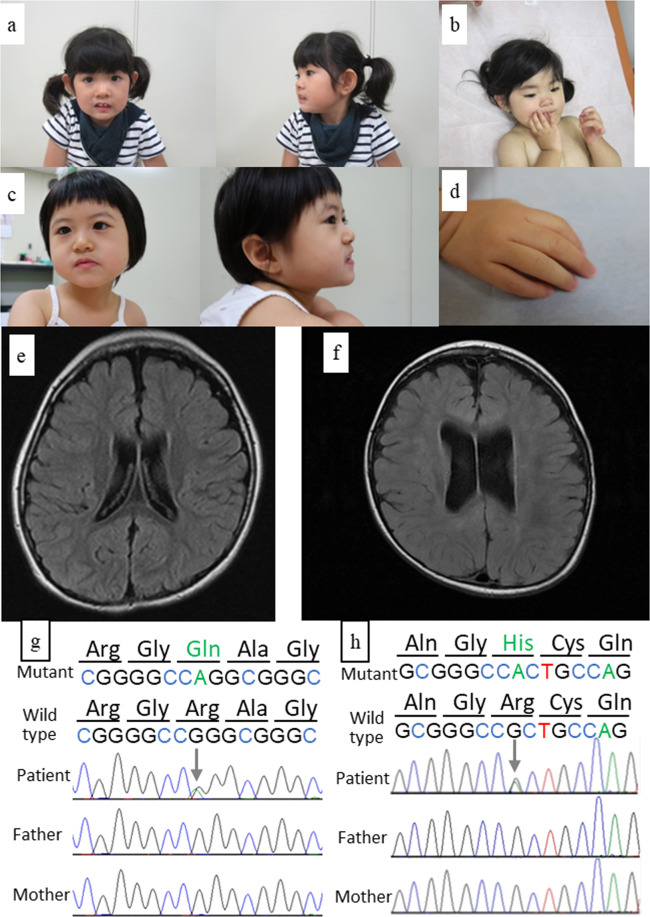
Patients with *DHX30* variants and their MRI findings. a Patient 2 at 3 years of age. The patient exhibited thick low-set ears, long eyelashes, interdental space, high arched palate, micrognathia, b tapered fingers and hand licking. c Patient 3 at 5 years of age. The patient exhibited internal strabismus, epicanthus, thick eye brow, long eyelashes, and d tapered fingers. e Axial T1-weighted brain MRI of Patient 2 demonstrated a decreased white matter volume and enlarged brain ventricles and extra-axial space. f Axial T2-weighted fluid-attenuated inversion recovery brain MRI of Patient 3 demonstrated significant decreases in the volume of the midbrain and ventricular dilatation and completed myelination with remaining multiple areas of patchy increased T2 signal around the cerebral white matter. *DHX30* variants were identified in patients 1 (g) and 2 (h) by the Sanger method. Arrows indicate variations.
